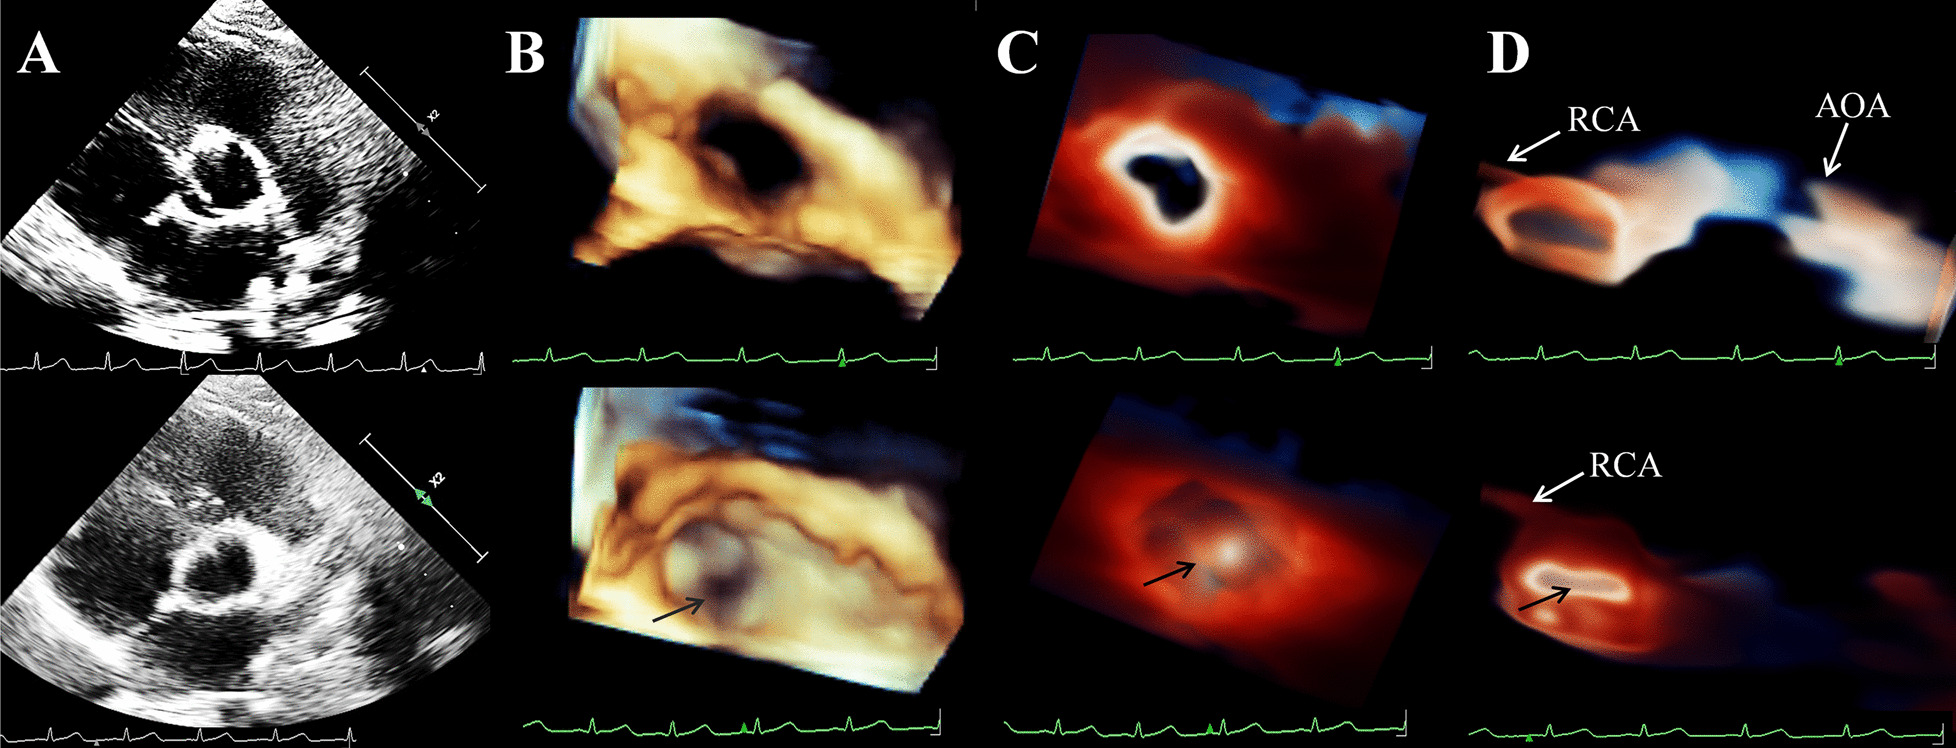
Bicuspid aortic valve. A 2D-TTE showing the aortic valve during systole (above) and diastole (below). B–D Traditional 3DE, TrueVue, and TrueVue Glass images demonstrating aortic valves when opened and closed, respectively. Black arrows show aortic valve closing line. *AOA* aortic arch, *RCA* right coronary artery, *2D-TTE* two-dimensional transthoracic echocardiogram, *3DE* three-dimensional echocardiography
